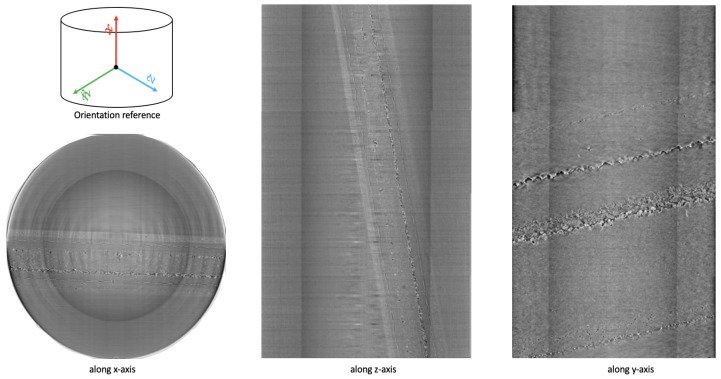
Sample raw data obtained after TomoPy reconstruction of a CT scan.
